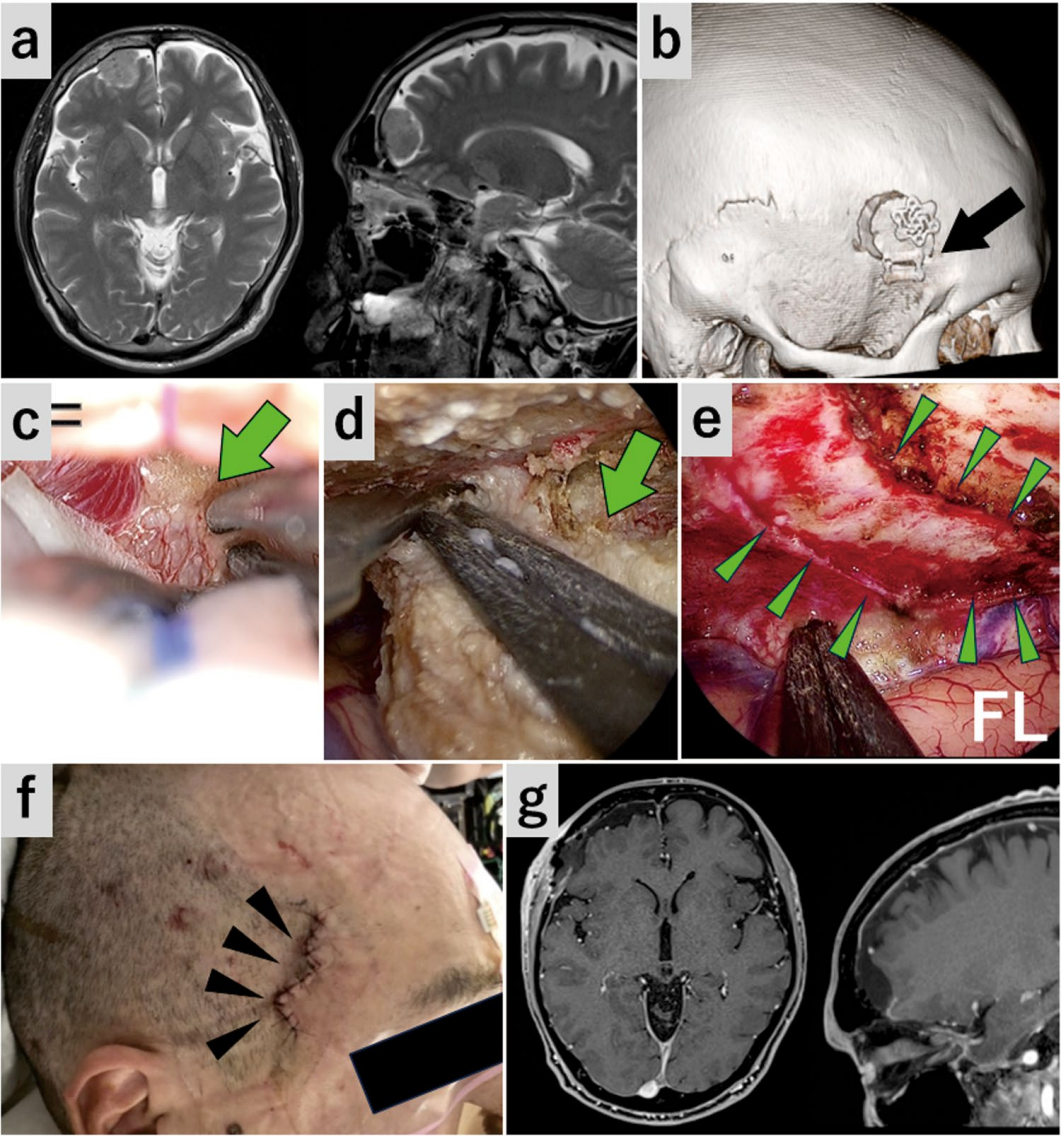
Case 3: Frontal meningioma (a) Preoperative T2-weighted MRI. b Postoperative 3D computed tomography shows craniotomy. Black arrow, ICP. c The lateral side of the tumor was excised under the exoscopic vision. Green arrow, tumor (d and e) The endoscope was inserted into the frontal subdural space. d The tumor was detached from the dura mater. Green arrow, tumor (e) The attachment dura mater was endoscopically resected. Duraplasty of the resected area was not necessary owing to the tight connection of the normal dura and the bone. Green arrowheads, cut margin of the dura mater; FL, frontal lobe. f Image immediately after surgery. Black arrowhead, W-shaped incision. g Postoperative gadolinium-enhanced T1-weighted MRI
